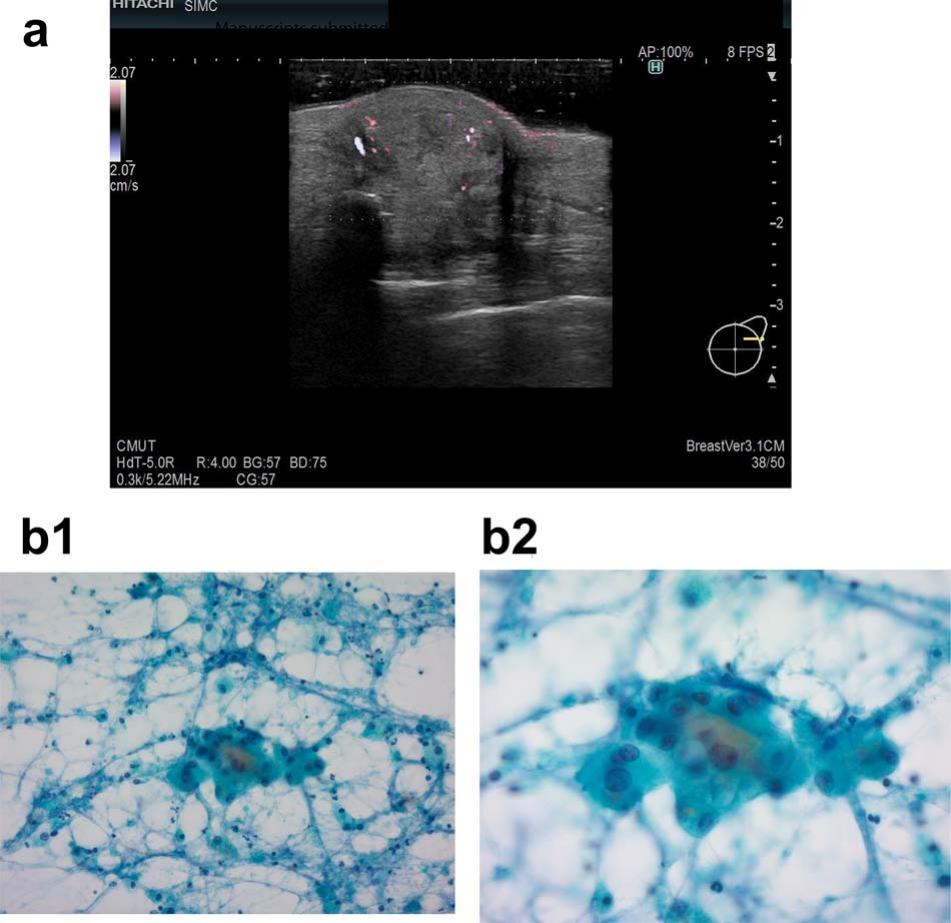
Ultrasound examination in case 2 revealed a mass 19.4 × 18.8 × 10.7 mm in the left breast (a) and cytodiagnosis did not unclear that it showed local recurrence. (b-1: ×20 magnification, b-2: ×40 magnification).
